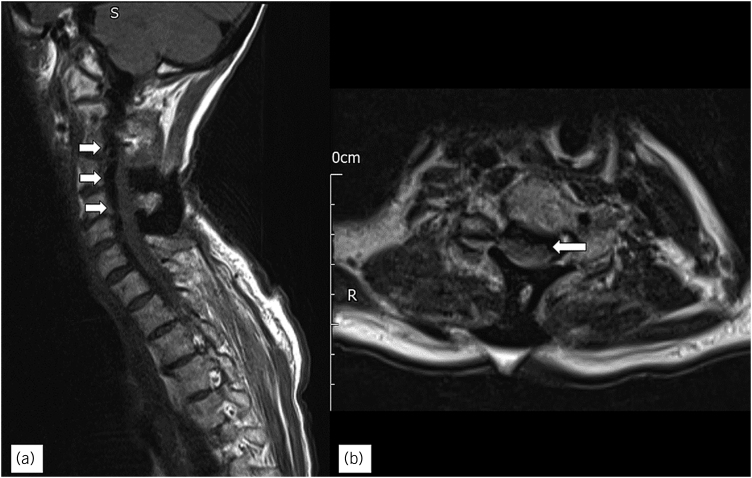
(a) T2 Sagittal MRI showing the ruptured collection communicating with the skin at the C4/5. Gas is tracking into the spinal canal (pneumorrhachis), and intracranially. (b) Communication of air with the spine (arrow). MRI = magnetic resonance imaging
